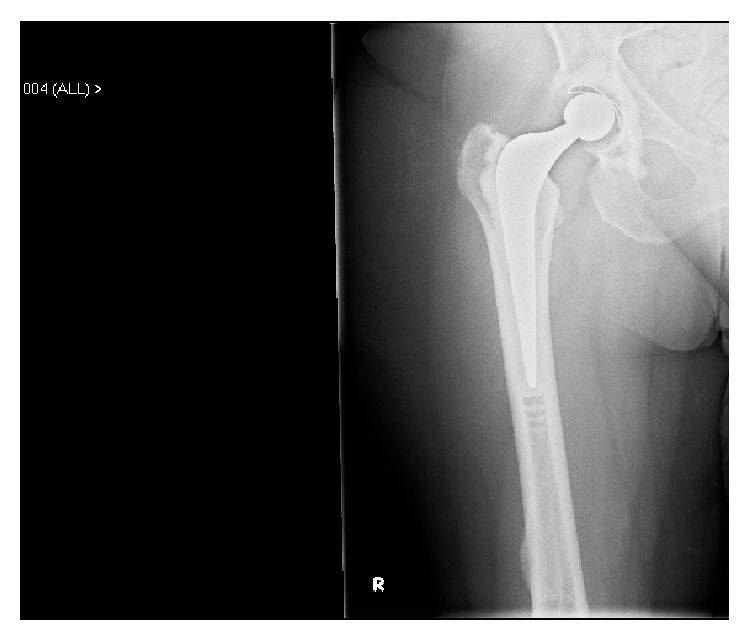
X-ray right femur showing callus formation.
